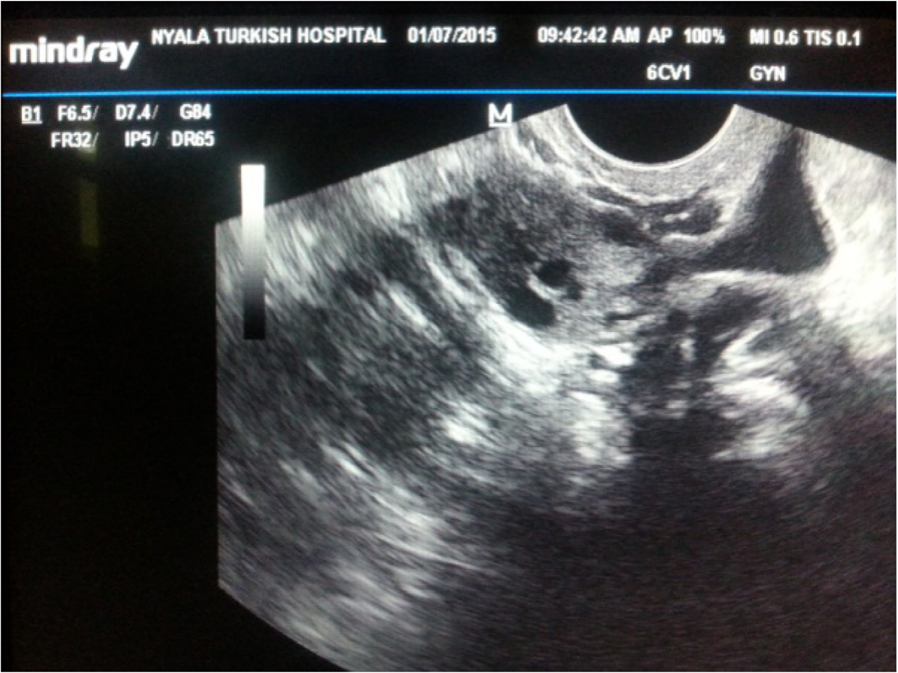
Control ultrasonographic image after the methotrexate treatment
